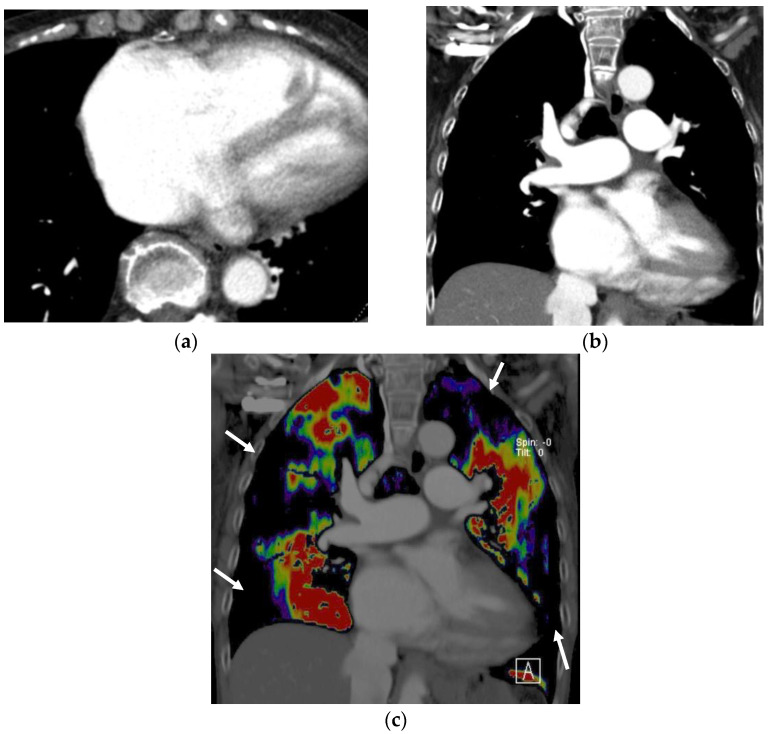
Images of the perfused blood volume in the lung of a patient with chronic thromboembolic pulmonary hypertension: (a) Transverse CT pulmonary angiography at the level of the heart demonstrates an enlargement in the right ventricle, which indicates right heart failure. (b) Coronal CT pulmonary angiography demonstrates dilatation of the main pulmonary artery. Conventional CT pulmonary angiography does not show information on lung perfusion. (c) The image of the perfused blood volume in the coronal lung, corresponding to image (b), demonstrates multiple perfusion defects (arrows) in the bilateral lung, which are typical findings of chronic thromboembolic pulmonary hypertension.
